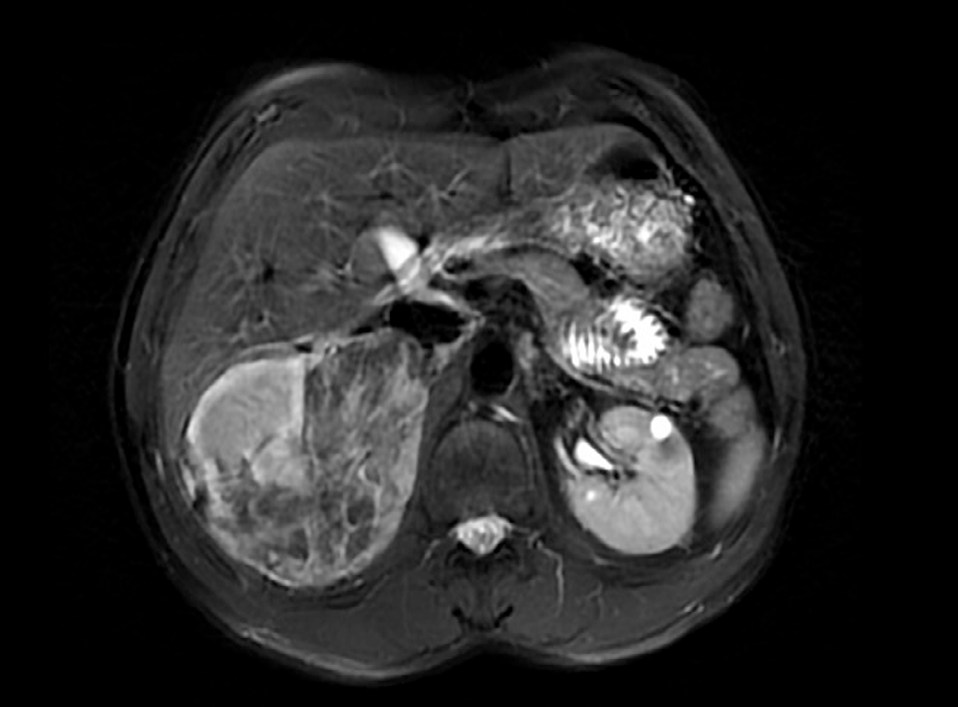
Bilateral multiple angiomyolipomas seen by magnetic resonance imaging in a 35-year-old male patient with tuberous sclerosis complex.
